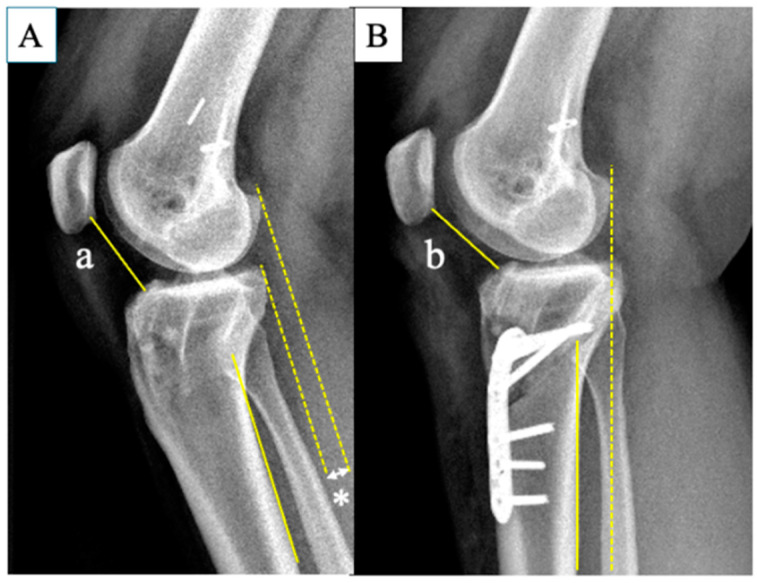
A representative case of a patient who underwent combined infratubercle ACWO with revision ACL reconstruction. (A) Preoperative plain lateral knee radiograph showing anterior tibial translation relative to the femur (*) due to ACL deficiency and an excessively high PTS. (B) Anterior tibial translation is reduced after surgery. Consequently, distance “a” increases slightly to distance “b” due to the reduction in anterior tibial translation after surgery. ACWO, anterior closing wedge osteotomy; ACL, anterior cruciate ligament; PTS, posterior tibial slope.
